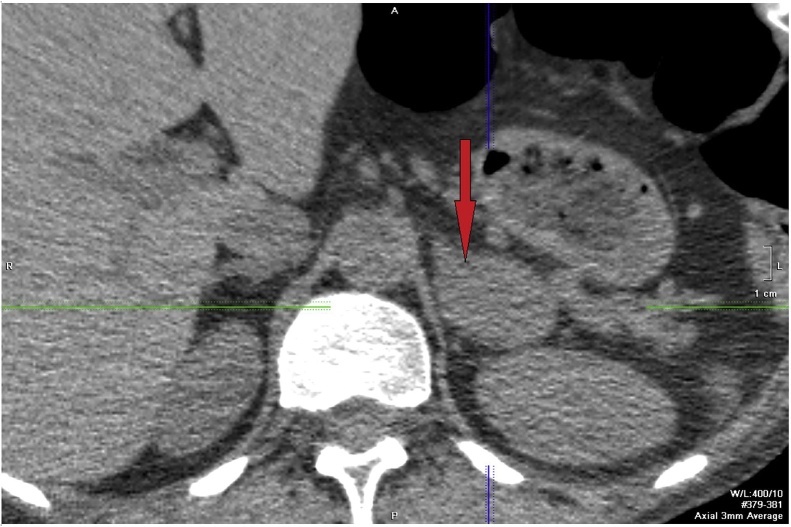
CT-imaging of unidentified mass.
